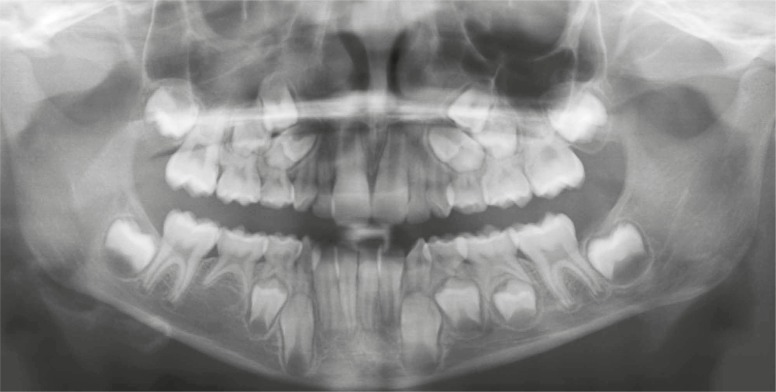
Initial panoramic radiograph showing bilateral maxillary permanent lateral
